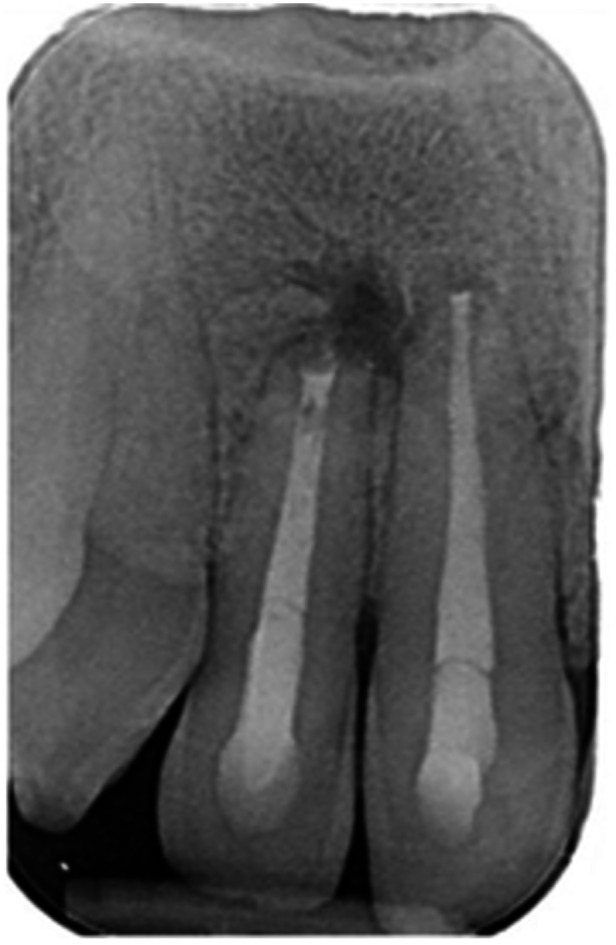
Case 9: The preoperative periapical radiograph reveals a large periapical radiolucency associated with tooth 12, extending to tooth 11. Apical surgery was performed on tooth 12 only. At the 6‐month follow‐up, complete PDL reattachment is observed on tooth 11, while tooth 12 shows incomplete healing. However, there is a significant reduction in the size of the periapical radiolucency.
